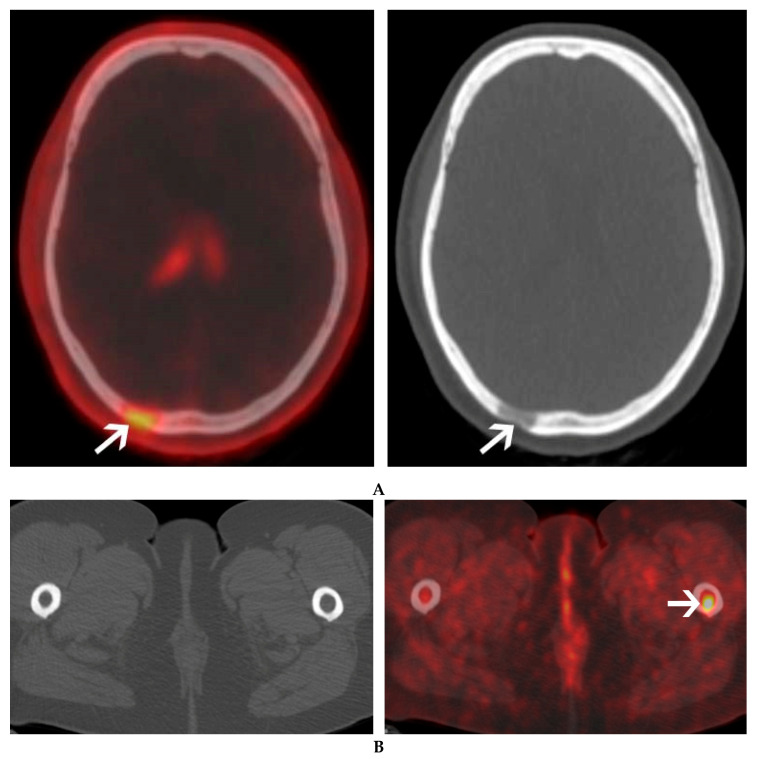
A 60-year-old female with IgG lambda smoldering multiple myeloma. An osteolytic lesion of the skull was found on a follow-up CT. ^18^F-Choline PET/CT was ordered to further characterize this lesion and search for additional bone lesions. ^18^F-Choline PET/CT axial image of the skull shows a moderate uptake of an occipital osteolytic lesion (A). Additional focal uptake of ^18^F-Choline was seen in the left femur, corresponding to a bone marrow lesion with no bone structural changes on CT (B).
